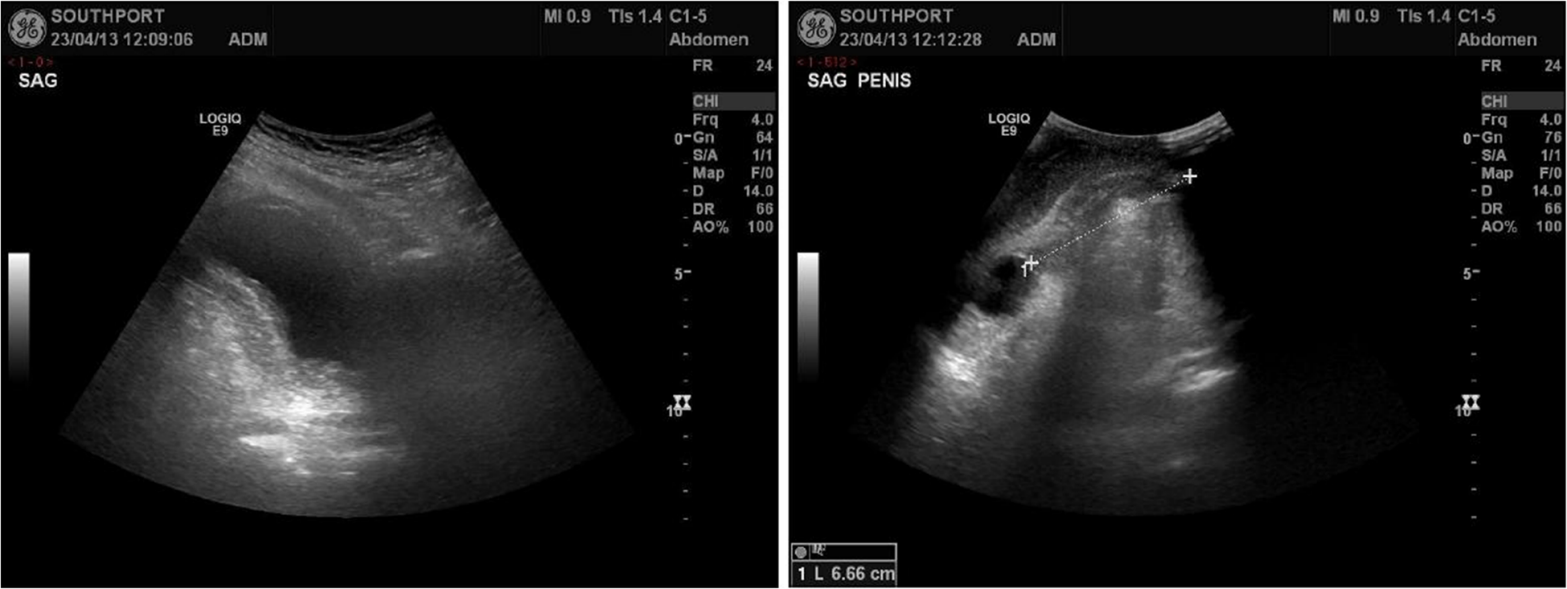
Top panel: Ultrasound scan of patient # 1 revealed no urinary catheter in the bladder. Bottom panel: The balloon of Foley catheter was seen in membranous urethra, 7 cm from the tip of penis.
